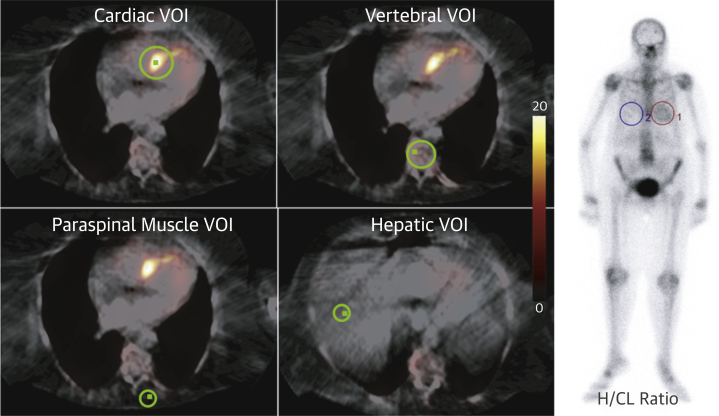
SPECT/CT and H/CL Ratio Quantification Fused axial single-photon emission computed tomography/computed tomography (SPECT/CT) ^99m^Tc-3,3-diphosphono-1,2-propanodicarboxylic acid images demonstrating volume of interest (VOI) (green) positioning for standardized uptake value (SUV) quantification on the left. The small green square within each VOI represents the peak SUV (SUV_peak_), which enables the reporter to ensure that the recorded SUV_peak_ lies within the desired organ/tissue. On the right, 3-h planar, whole-body ^99m^Tc-3,3-diphosphono-1,2-propanodicarboxylic acid image of a different patient with regions of interest over the heart (1) and contralateral lung (4). H/CL = heart/contralateral lung.
